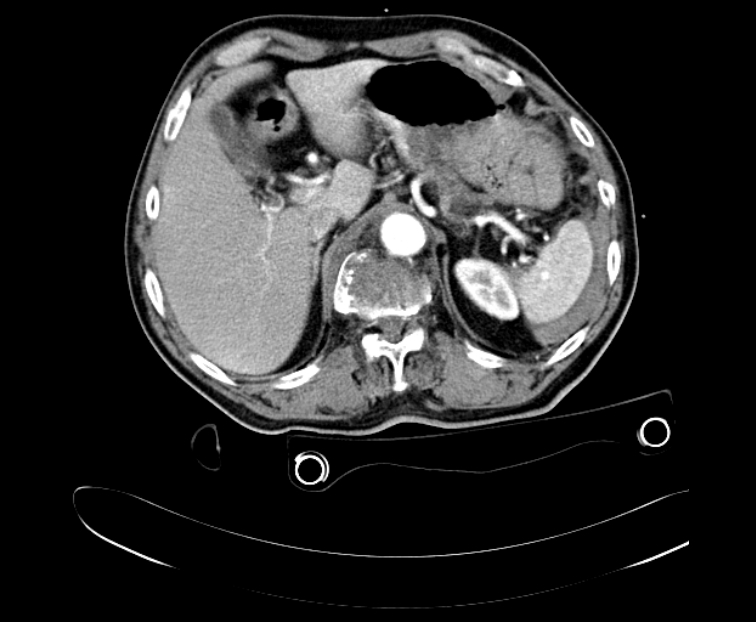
CT scan showing contrast blush in grade II splenic injury which was later confirmed by surgery; perisplenic hemoperitoneum.
